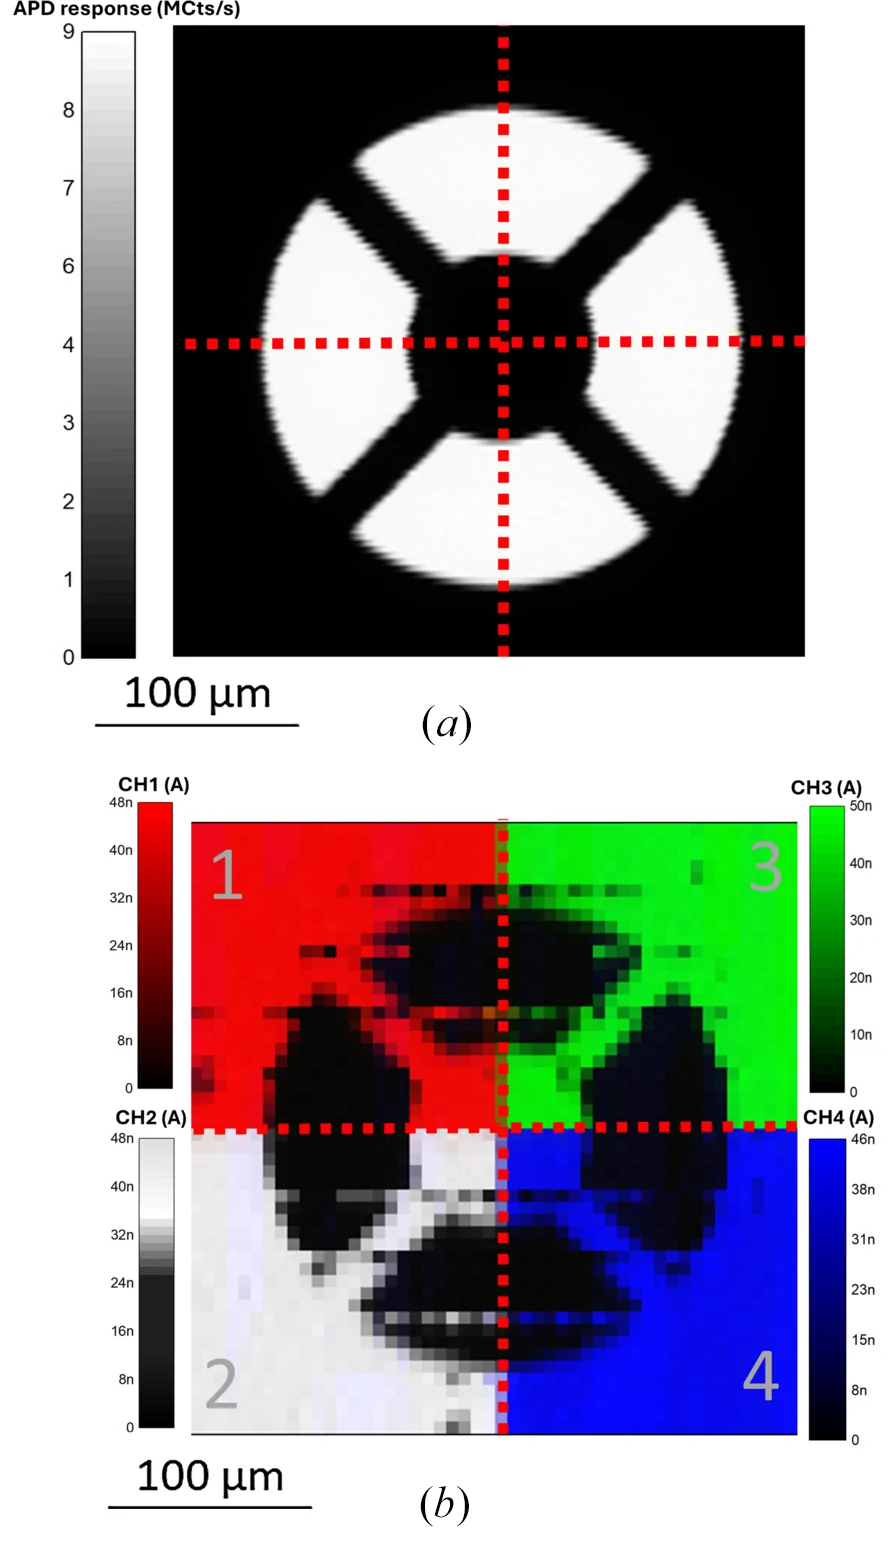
(*a*) STXM image of the X-ray beam illuminating the SiC CS shown in Fig. 4 ▸. (*b*) Response of the four individual sectors of the SiC diode [marked in (*a*)] to the focused X-ray beam illuminating the section of the diode.
